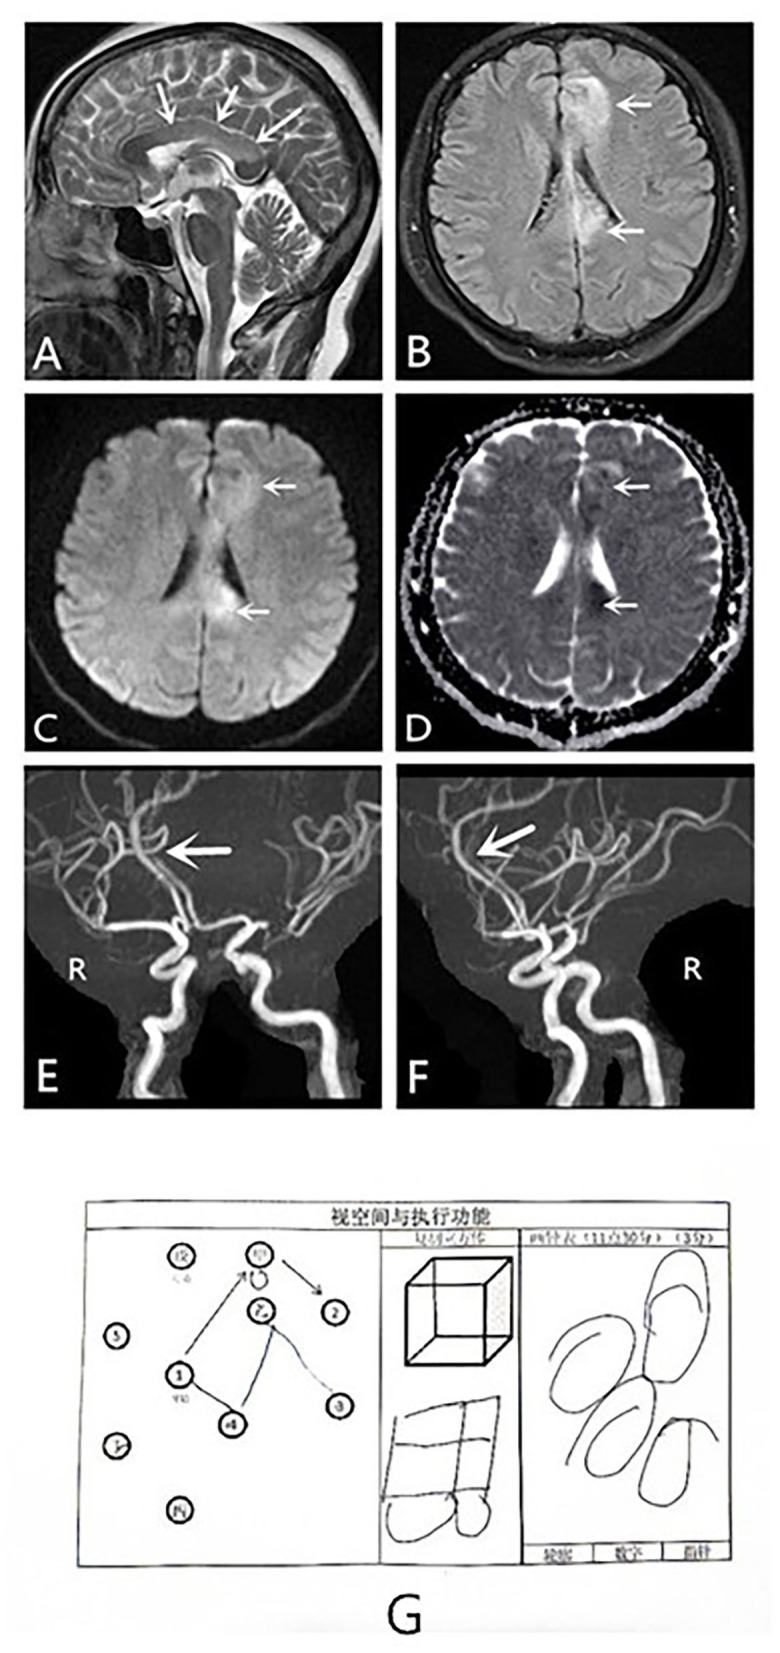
MRI, MRA and visuospatial and executing skills of the dominant hand results of a patient with alien hand syndrome. MRI: (A–D) Sagittal T2WI scan, axial T2 FLAIR, DWI, and ADC scans show cytotoxic edema in the left frontal lobe and the body of the corpus callosum. (E,F) MRA shows poor visualization of the pericallosal artery and stenosis of the left middle cerebral artery. The patient failed to recognize spatial structure, known as constructional apraxia (G). ADC, apparent diffusion coefficient; DWI, diffusion-weighted imaging; FLAIR, fluid-attenuated inversion recovery; MRA, magnetic resonance angiogram; MRI, magnetic resonance imaging; T2WI, T2-weighted imaging.
